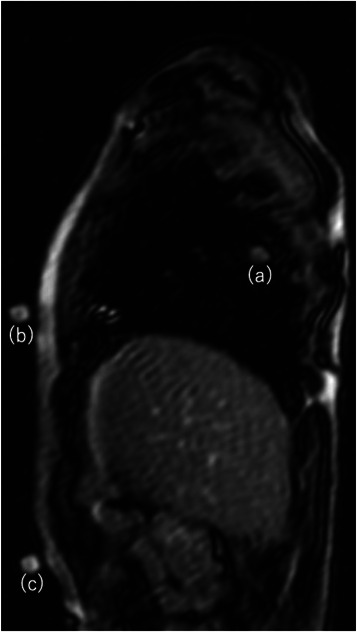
Cine MRI. (a) is the target and (b) and (c) are body surface markers. When the cine MRI was taken, multiple markers were attached, but when creating the waveform, a single marker was selected and the waveform was created.
